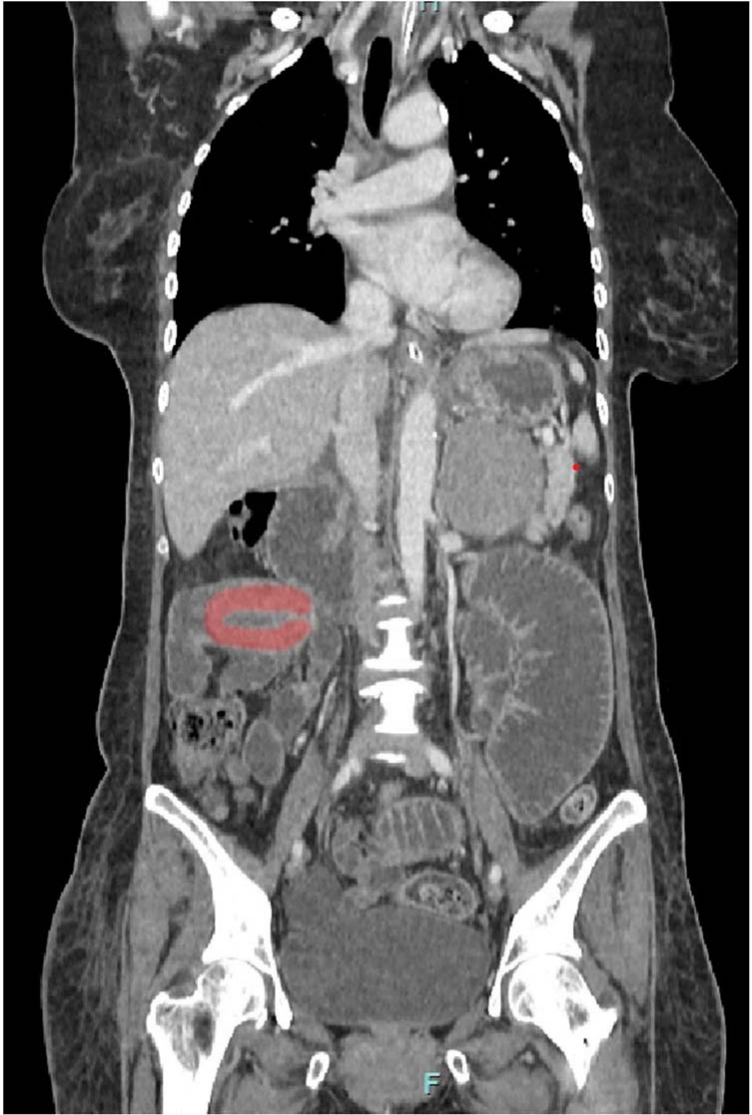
Coronal view of CT scan. Intussusception transition point is seen likely at the proximal ileum with evidence of ‘bowel in bowel’ appearance, measuring ~7.8 cm.
